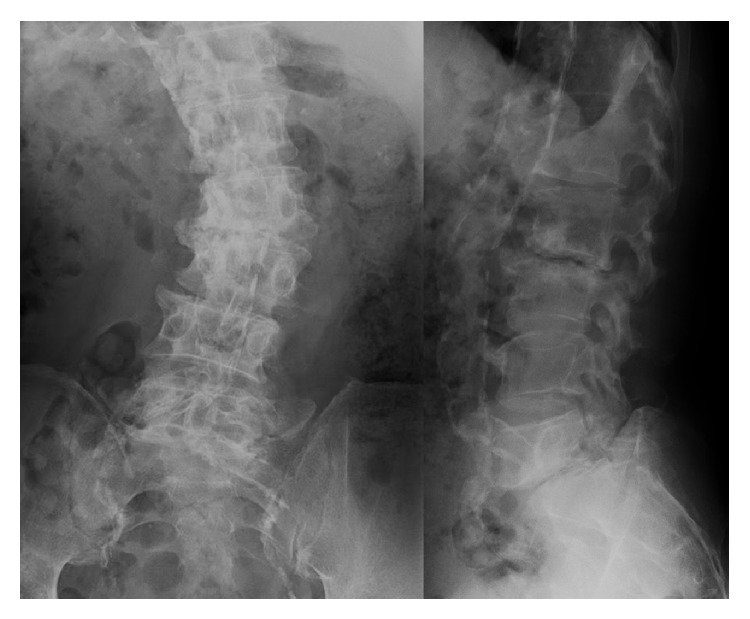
Preoperative plain radiographs showing progression of scoliosis and destructive changes at L2-L3 and L5-S1.
